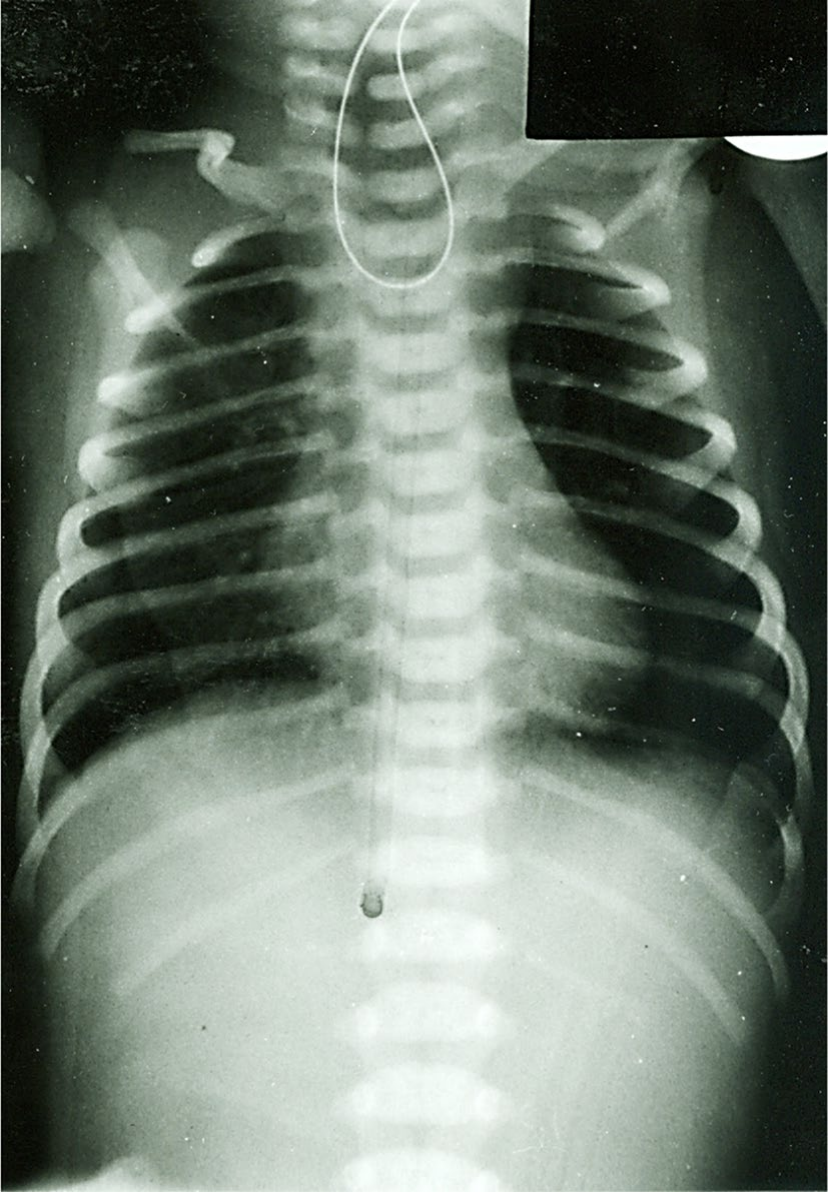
Plain X-ray of a newborn with LGEA showing the radiopaque gastric tube in the blind-ending upper esophageal pouch and no air below the diaphragm (“gasless abdomen”)
